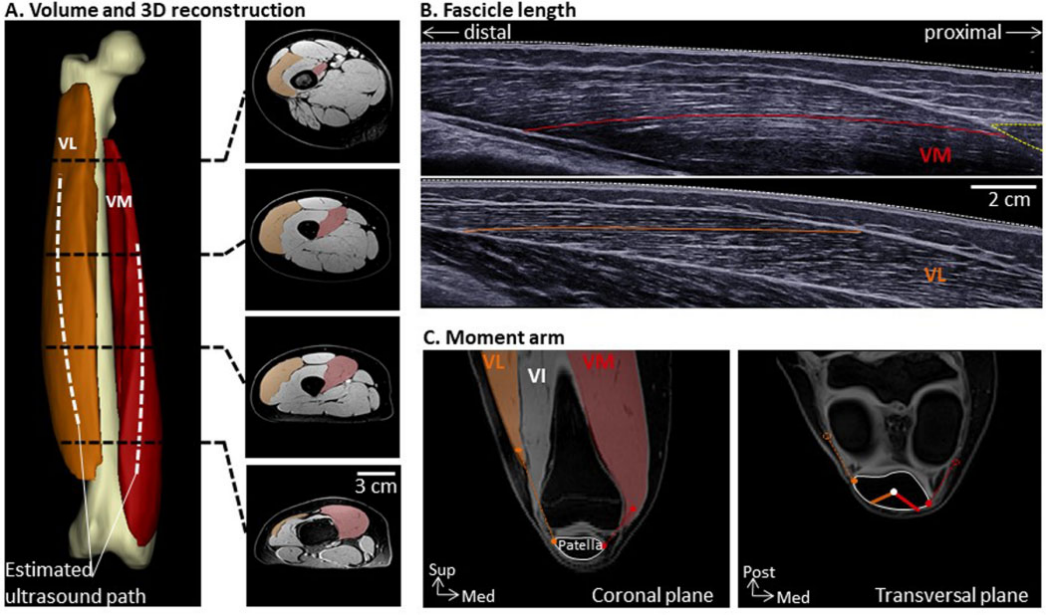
Experimental measures of physiological cross-sectional area (PCSA) and moment arm for vastus medialis (VM) and vastus lateralis (VL). (A) VM, VL, and femur 3-dimensional (3D) reconstruction from the magnetic resonance imaging acquisitions. VM and VL were manually segmented from each axial image. Their volumes were reconstructed using 3D image analysis software. Example of cross-sectional images are taken from 20%, 40%, 60%, and 80% of femoral length. (B) Fascicle length was determined from panoramic ultrasound images of VM and VL using a custom-made MATLAB script. Examples of 1 fascicle from each muscle are delineated. The yellow dotted line on the VM image indicates the sartorius, which was the anatomical landmark used to determine the probe’s line of travel on the skin for VM. The PCSA of each muscle was calculated as its volume divided by fascicle length. (C) VM and VL moment arms were defined as the perpendicular distance from the line of action of their respective tendon to the patellar centroid. Lines of action are shown in dashed lines. Two-dimensional projections of the VM and VL moment arms are shown by thick lines on the transverse view. Med, medial; Post, posterior; Sup, superior; VI, vastus intermedius.
